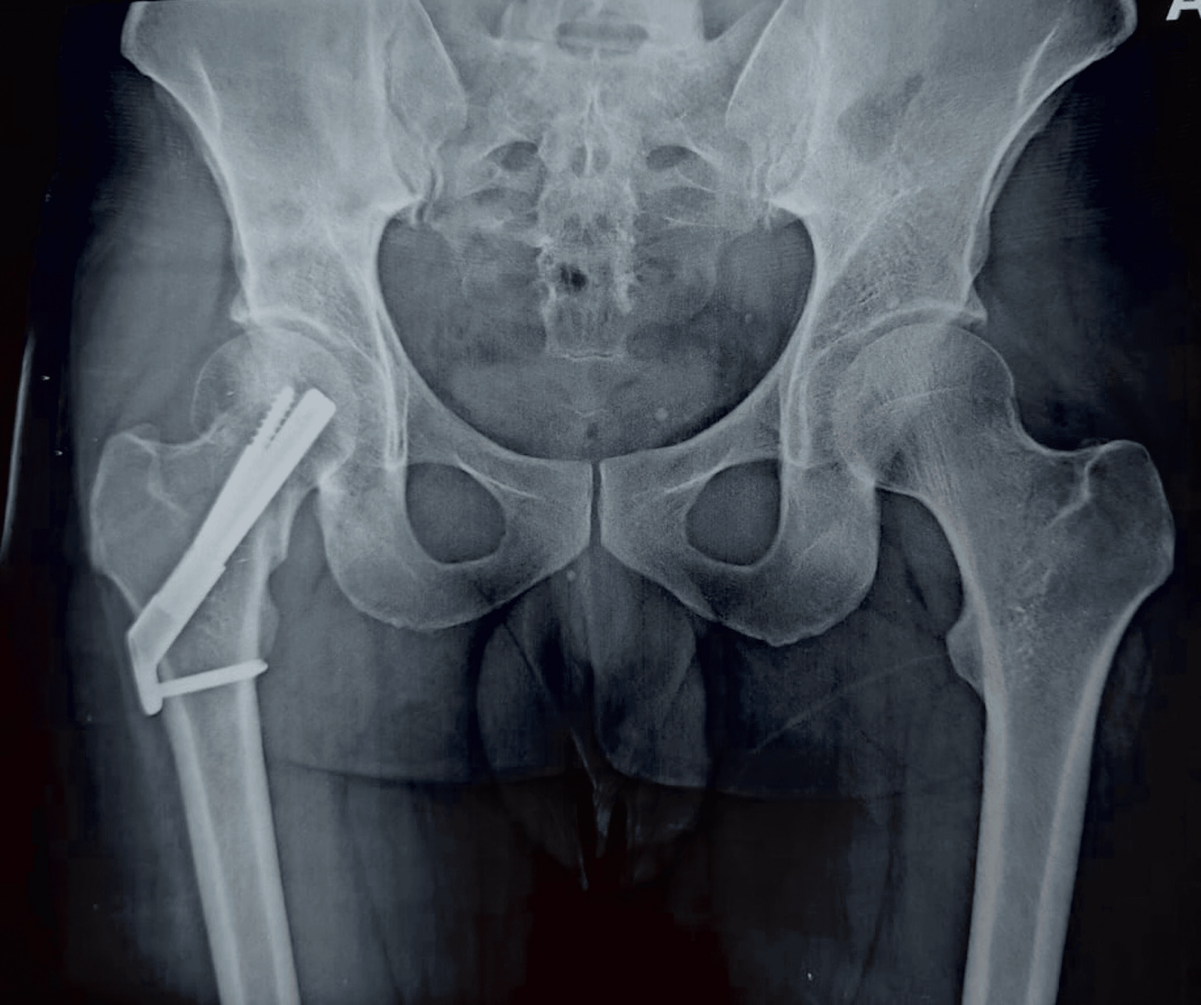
Immediate postoperative X-ray of the pelvis and both hips in an anteroposterior view showing the Femoral Neck System in situ.
